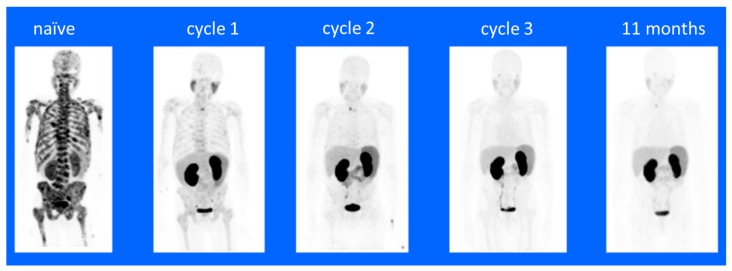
^68^Ga-PSMA-11 PET/CT images of a treatment-naïve patient with extensive bone metastasis at primary diagnosis. A complete remission was observed after three cycles of ^225^Ac-PSMA-617 with de-escalating activities of 8/7/6 MBq. The patient remained symptom-free with undetectable serum PSA and a negative ^68^Ga-PSMA-11 PET/CT at 11-month follow-up evaluation. This figure and legend were reproduced from Sathekge, et al. [6].
